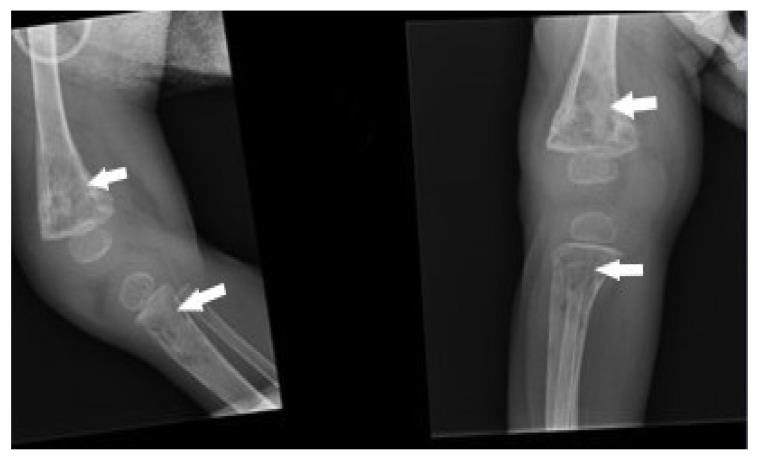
Radiographic image of bone destructive changes in distal metaphysis of the femur and proximal metaphysis of the tibia (white arrows).
